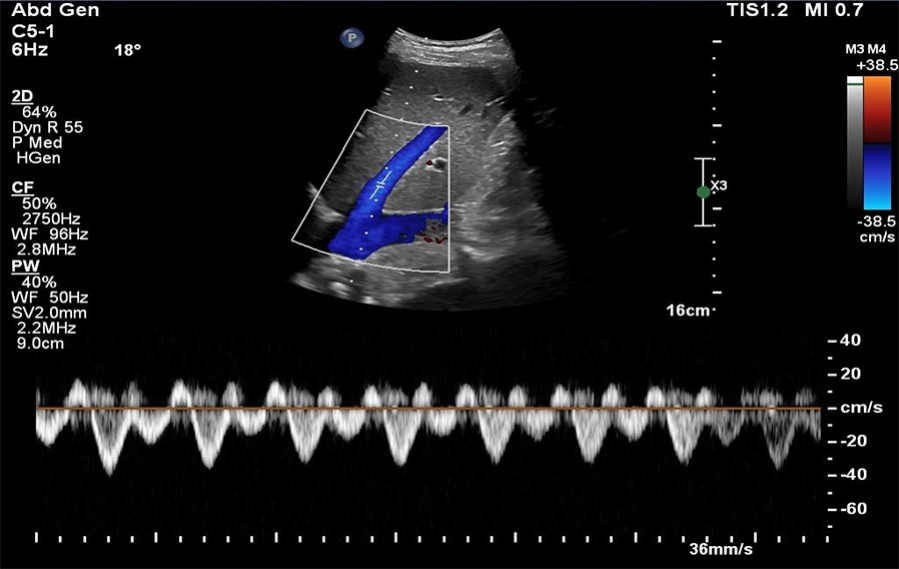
Pulsed-wave Doppler tracing of the HV in abdominal preset. HV, hepatic vein
